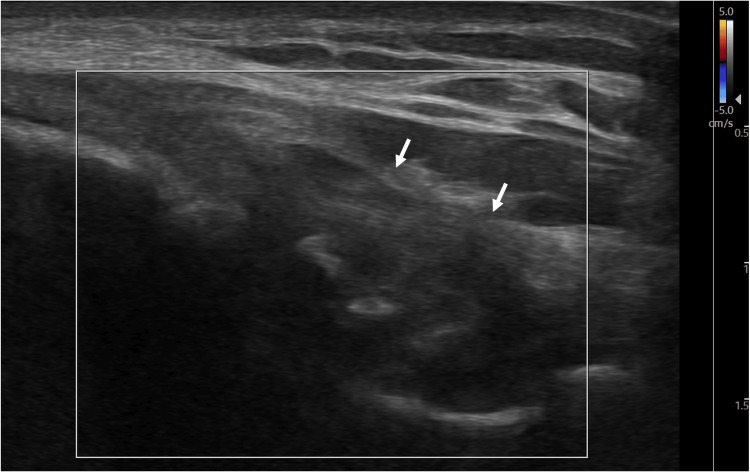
Long-axis view of the UCL with the color Doppler tool not visualizing any hyperemia. Arrows: Ulnar collateral ligament (UCL).
